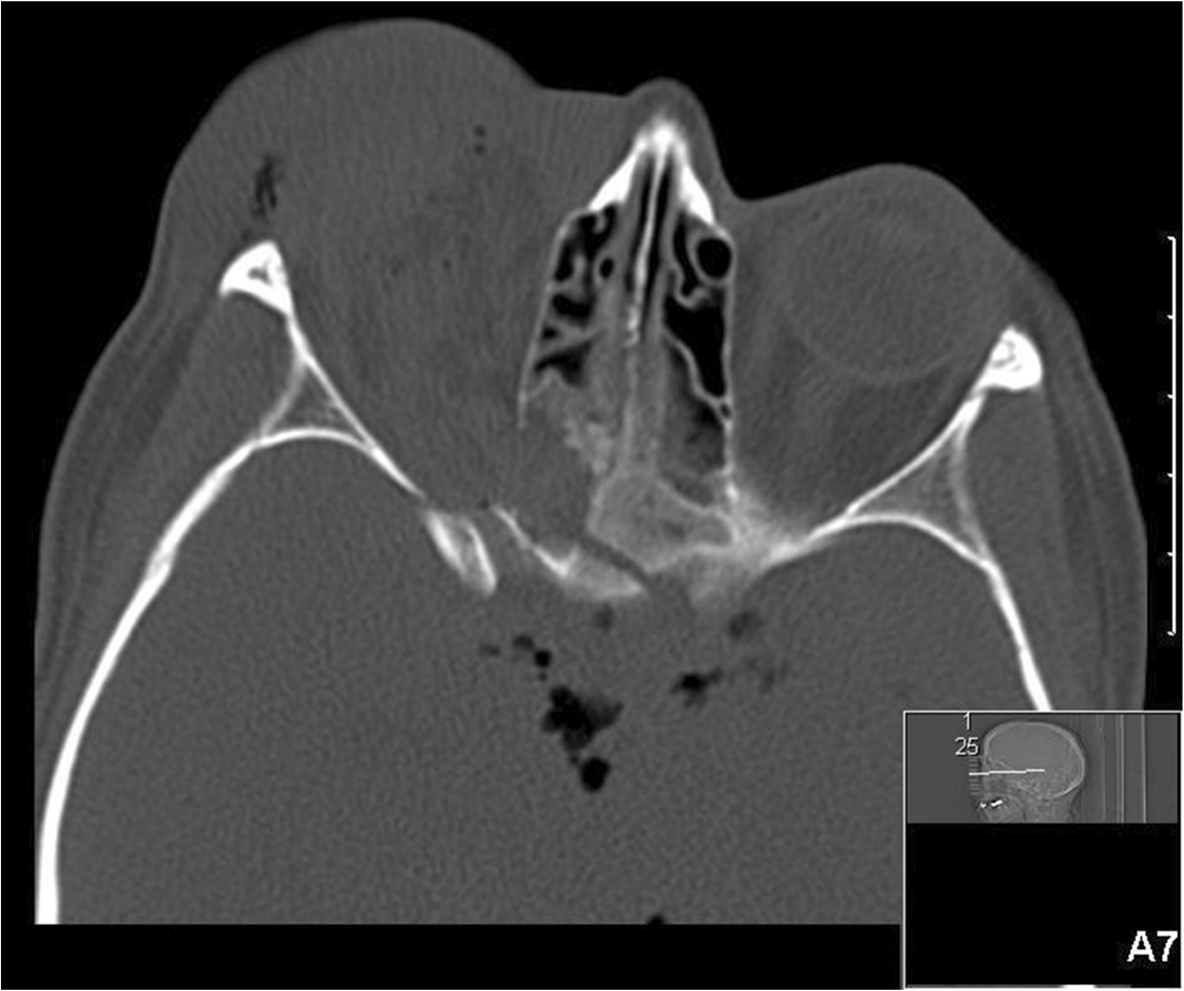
CT showing a left orbital roof fracture and pneumocephalus.
